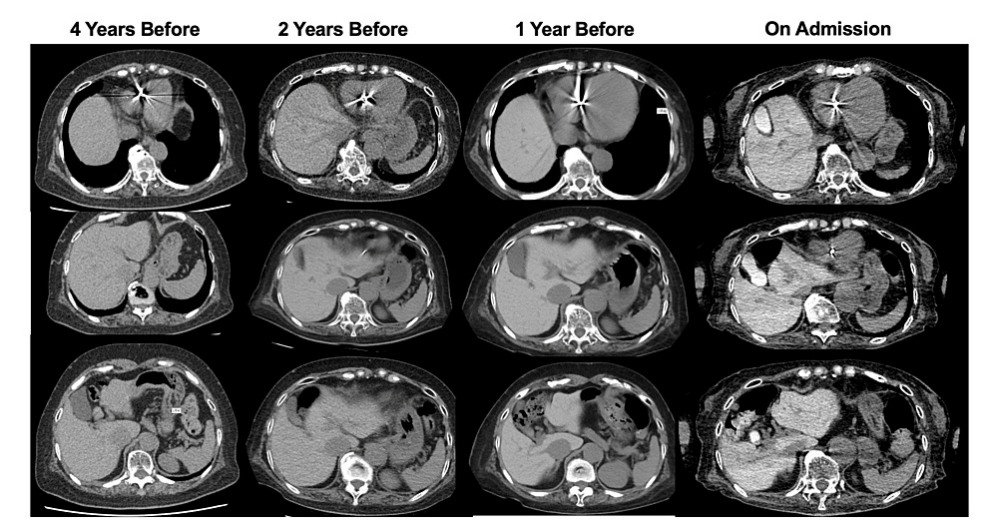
Abdominal non-contrasted CT The non-contrast CT scan of the abdomen at the time of admission revealed diffuse hyperattenuation within the liver parenchyma and the bile in the gallbladder. There was a noted gradual increase in the liver's attenuation when compared to previous CT scans. The mean liver attenuation values were 70 Hounsfield Units (HU) four years before, 81 HU two years before, 99 HU one year before, and 134 HU at the time of admission.
